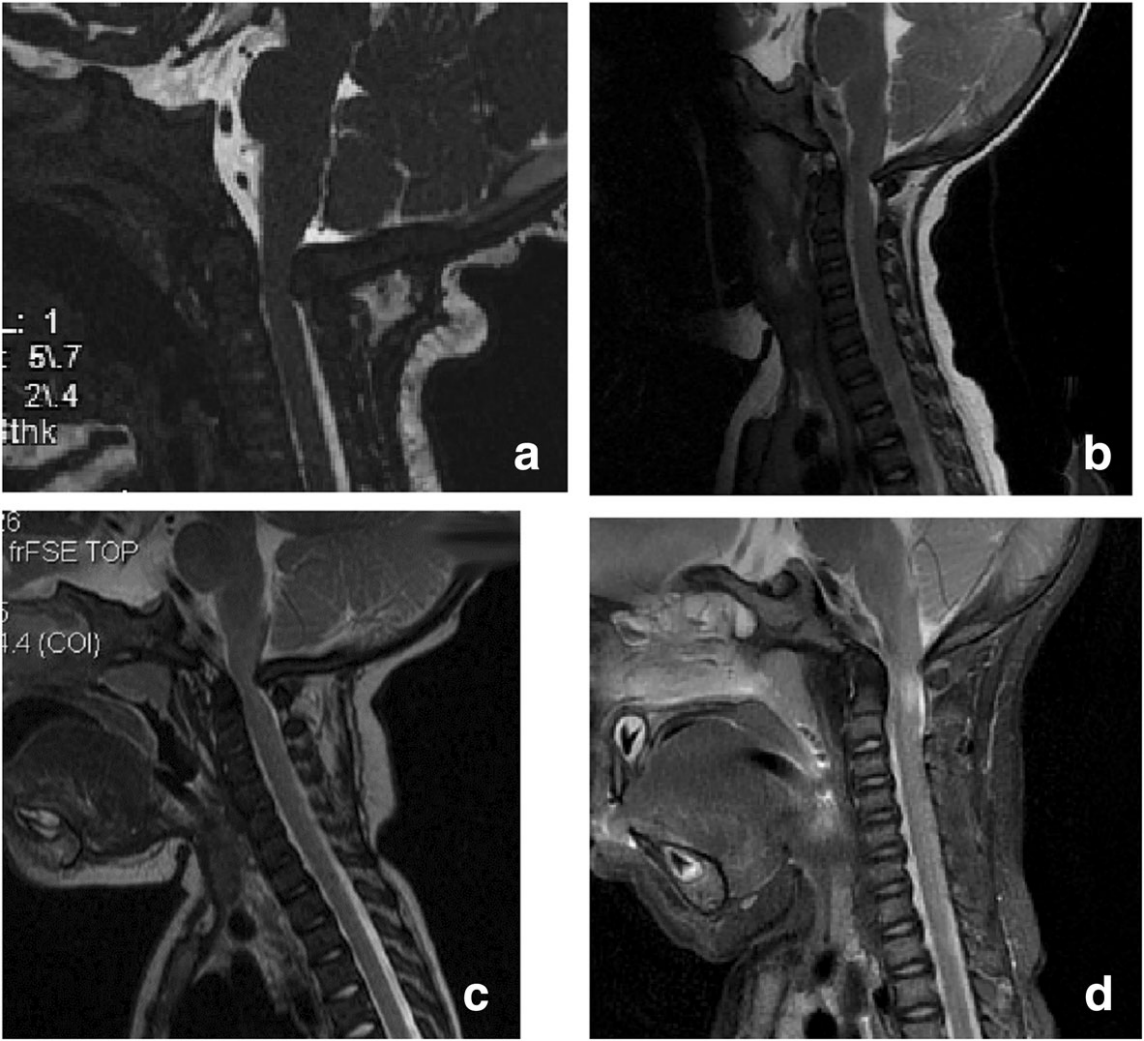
Sagittal views of magnetic resonance imaging of the cervical cord in four infants with achondroplasia. a There is obliteration of the posterior subarachnoid fluid layer; b In addition to obliteration of the fluid layer posteriorly, there is “nicking” of the posterior cord; c Narrowing of the cord is evident secondary to “waisting” – that is, there is some indentation anteriorly, as well; d An obvious T2 signal abnormality is present
